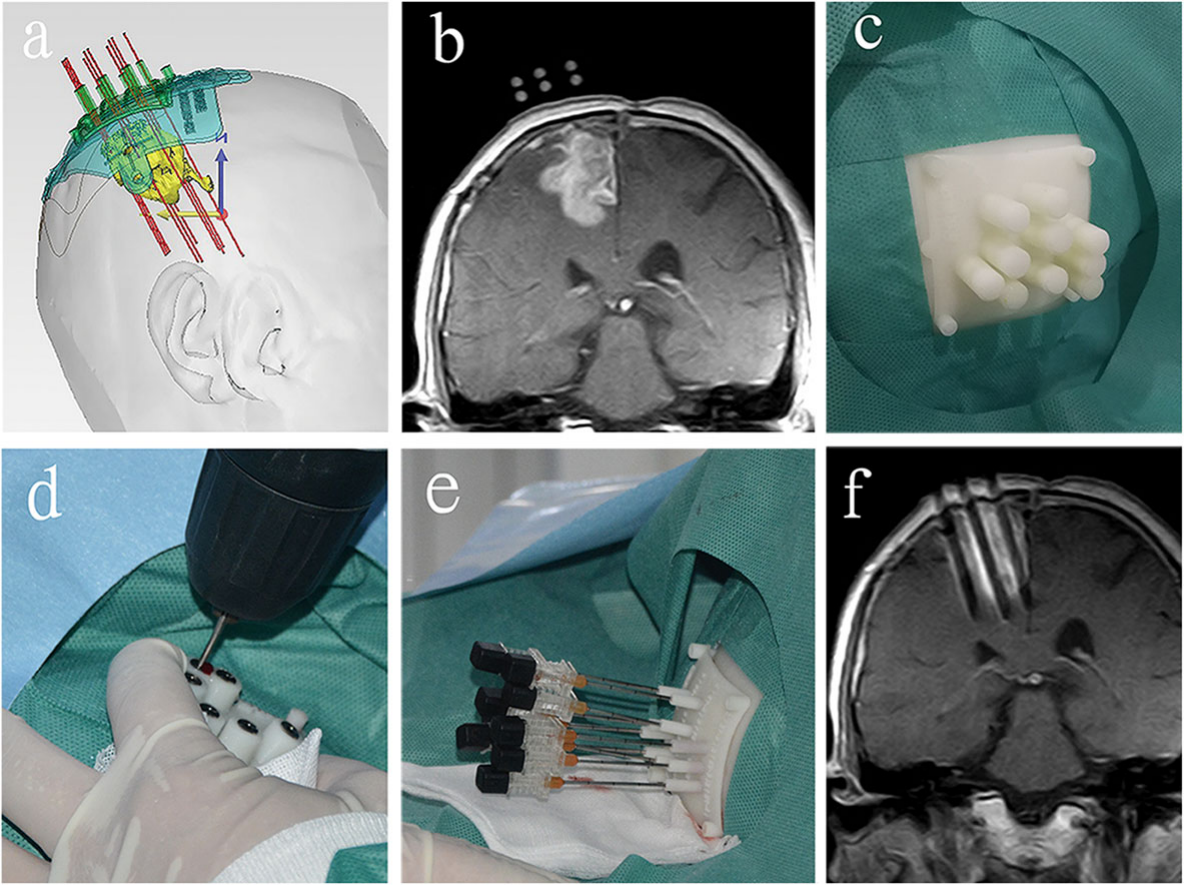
The procedure of 3DNPT combined with MR-guided ^125^I brachytherapy for recurrent glioblastoma. This patient had undergone surgery, radiotherapy, and temozolomide treatment before brachytherapy. a Preoperative design of 3DNPT. b The markers in the positioning template and the tumor were displayed in MRI. c The positioning template was placed and fixed on the scalp. d Twist-drill holes were made through drilling template. e Needles were punctured into the tumor through the puncturing template. f The position of needles could be displayed by the inoperative MRI
